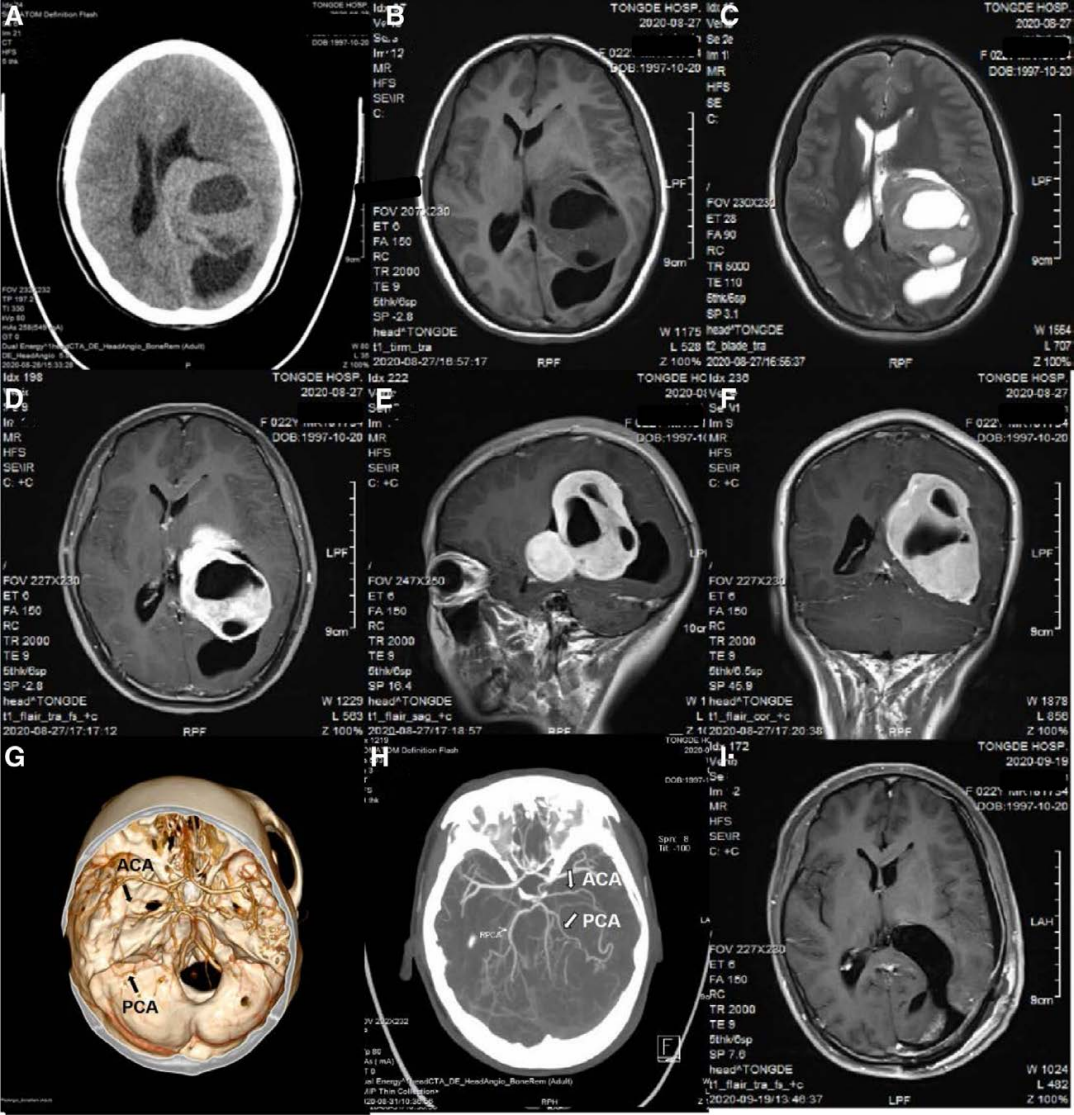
(A–I) Radiographic features of case 2 in the perioperative period. (A) CT scans revealed an extremely large mass in the left lateral ventricle with intratumoral multiple low-density cystic lesions. (B–F) MRI scans showed similar imaging features of the tumor on T_1_ weighted sequence, T_2_WIs, and the contrast-enhanced T_1_WIs following the injection of a contrast medium. (G and H) CTA indicated an affluent blood supply stemming from ACA and PCA. (I) Postoperative contrast-enhanced T_1_WIs demonstrated total resection of the tumor. ACA = anterior choroidal artery, CT = computed tomography, CTA = computed tomographic angiography, MRI = magnetic resonance imaging, PCA = posterior choroidal artery, T_1_WIs = T_1_ weighted sequence, T_2_WIs = T_2_ weighted sequence.
